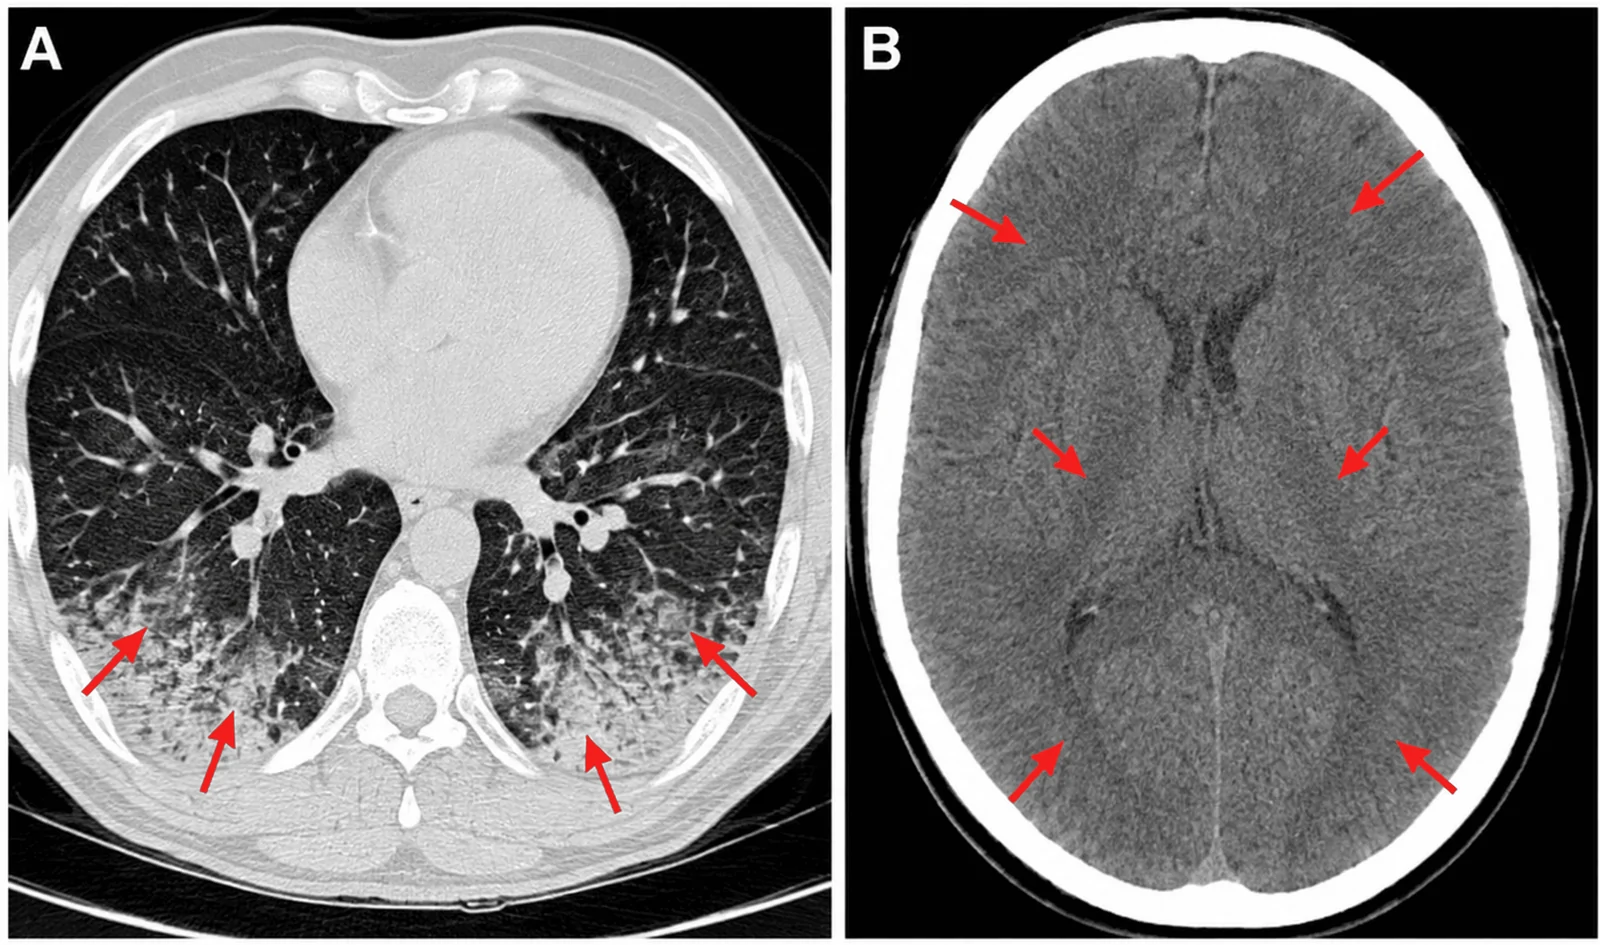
Thoracic and cranial CT A: Axial thoracic CT of the lung window demonstrates bilateral posterior-basal consolidative and ground-glass opacities (red arrows) in the dependent portions of both lower lobes, compatible with aspiration-related pulmonary involvement. B: Axial non-contrast cranial CT shows diffuse loss of gray-white matter differentiation, effacement of cortical sulci, and narrowing of the lateral ventricles (red arrows), findings suggestive of global hypoxic-ischemic cerebral injury following cardiopulmonary arrest.
